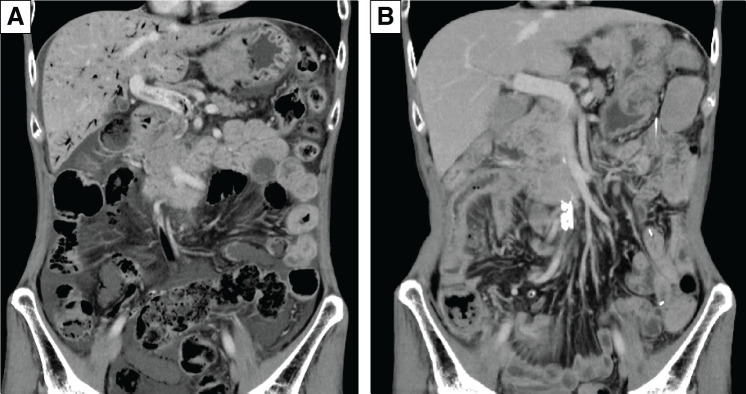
CT of the first occurrence of PI and PVG. Contrast-enhanced CT revealing extensive PI involving the entire length of the small intestine, and prominent gas extending from the mesenteric veins to the portal vein, with no evidence of free air or areas of poor contrast enhancement in the intestines (A). Five days after initiating chemotherapy, the patient’s abdominal symptoms and overall condition rapidly improved, and both PI and PVG signs were no longer visible on CT (B). PI, pneumatosis intestinalis; PVG, portal venous gas
